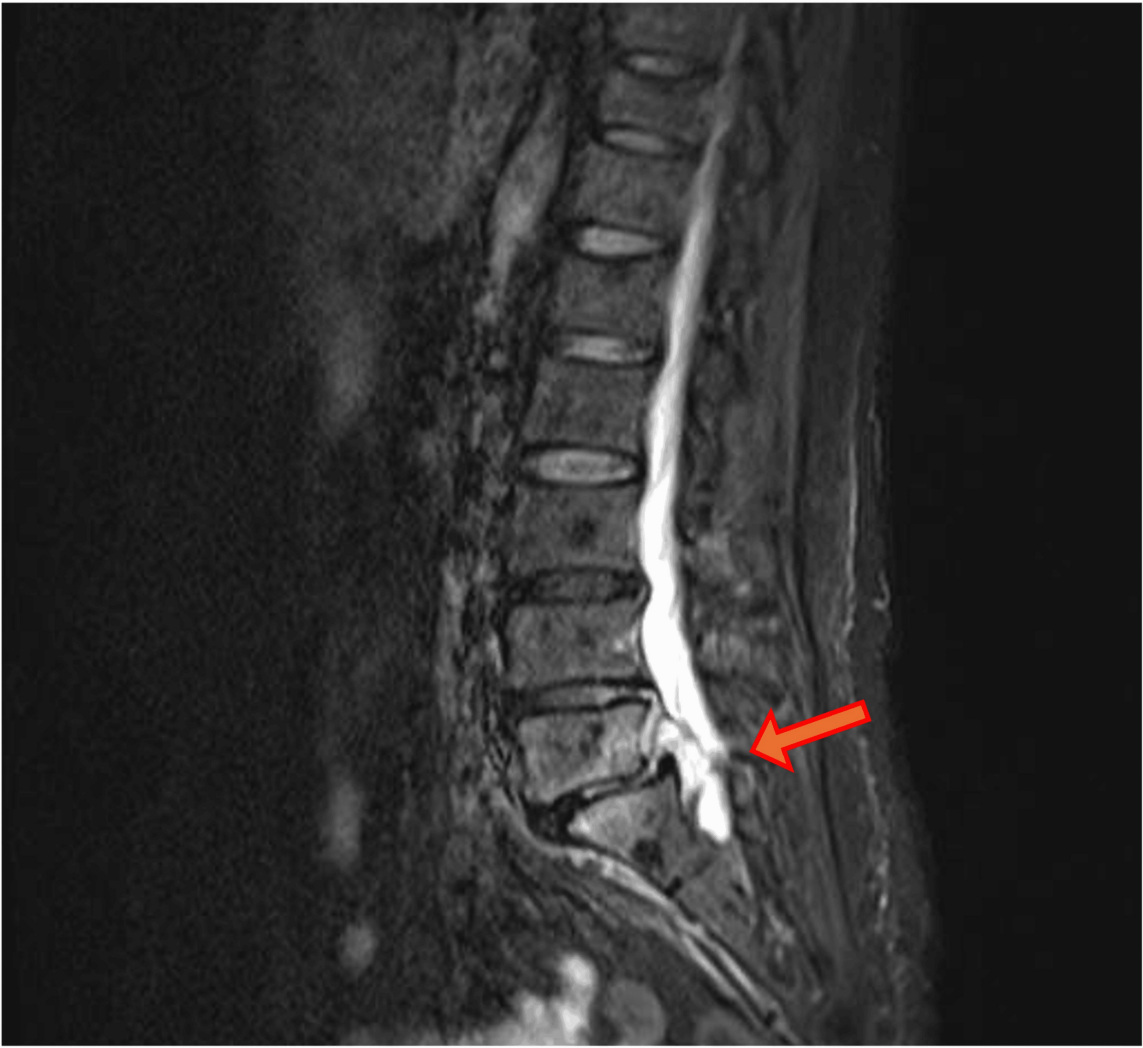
The MRI STIR at her second admission On the MRI STIR at the second admission, discitis at L5/S1 and a left-sided epidural abscess at S1 (red arrow) were observed. STIR: short tau inversion recovery
